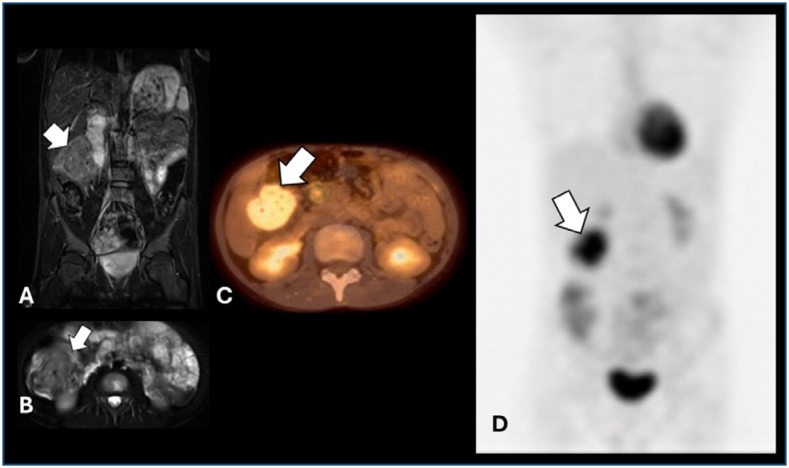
(A–D) 16-year-old boy with colon adenocarcinoma: coronal (A) and axial short tau inversion recovery images (B) of whole-body magnetic resonance imaging through the abdomen demonstrate heterogeneous hyperintense mass in the right hemicolon (white arrows). Positron emission tomography-computed tomography fusion axial image (C) indicates increased Fludeoxyglucose F 18 uptake (white arrow) in the mass. Positron emission tomography-computed tomography metabolic map image (D) shows Fludeoxyglucose F 18 uptake compared to surrounding tissues.
